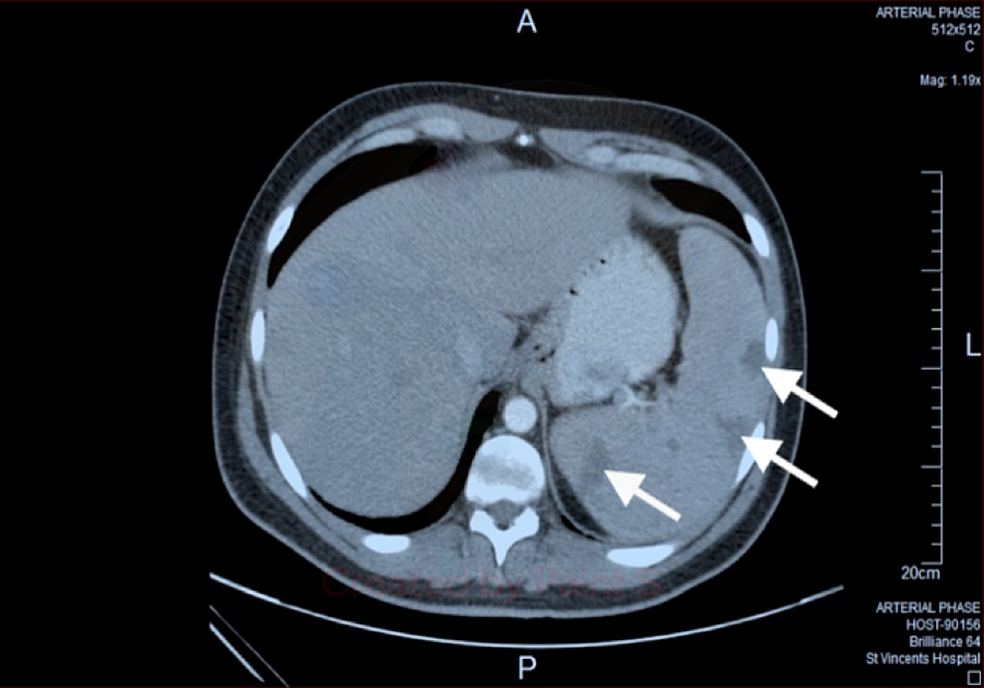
An abdominal CT scan performed eight days after admission showed splenomegaly with peripheral wedge shaped splenic infarcts.
